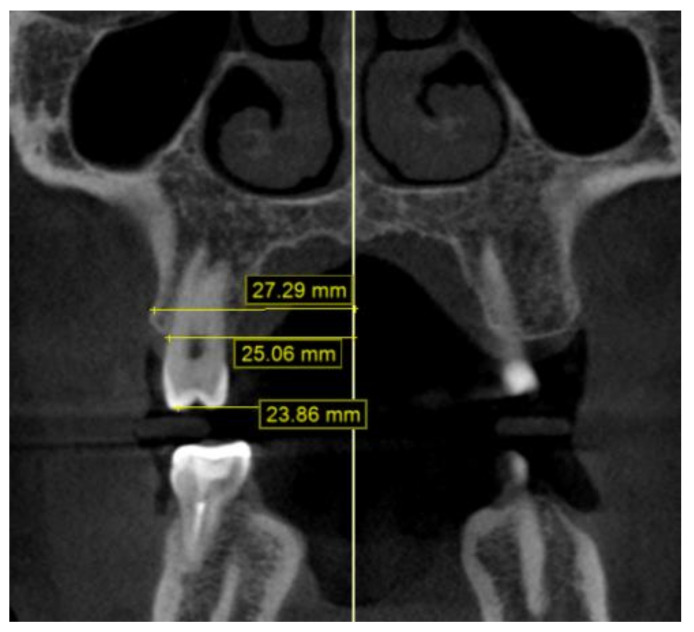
Distances from second premolar to medial sagittal plane on CBCT scan: Walaridge, gingival buccal level, and buccal cuspid.
